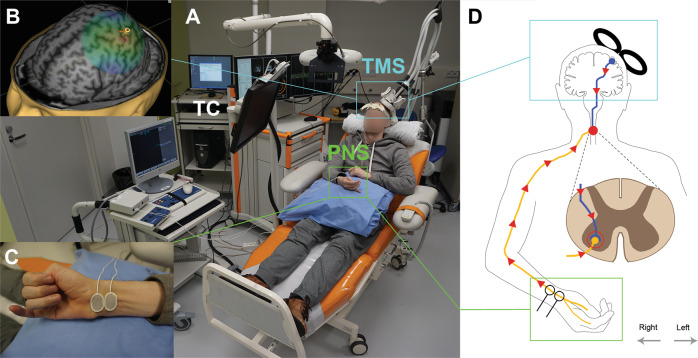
a Stimulation setup for long-term PAS therapy, b, c stimulation sites, and d basic principle of PAS. The upper motor neurons in the left primary motor cortex were stimulated with the TMS coil placed over the subject’s head (marked with blue squares). The electric field and a stimulated hotspot in the brain were visualized online using a 3D MRI-based model (b) that ensures accuracy and repeatability of stimulation. c High-frequency electrical stimulation was delivered to a peripheral nerve of the contralateral hand (marked with green squares). d The interstimulus interval was adjusted for collision of multiple descending volleys propagating along the upper motor neurons within the corticospinal tract (dark blue line) and ascending volleys travelling along the lower motor neurons within the peripheral nerve (yellow line) at the level of their synaptic contacts (red circle) in the cervical segment of the spinal cord (shown in enlarged form in d)
